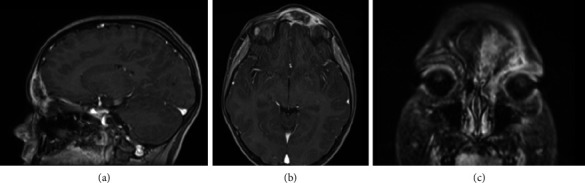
Pretreatment maxillofacial MRI ((a) sagittal view, (b) axial view, and (c) coronal view) showing a left frontoethmoidal lesion involving the orbital roof, measuring 25 × 20 mm, with inhomogeneous contrast enhancement, affecting the posterior wall of the frontal sinus and the orbit.
